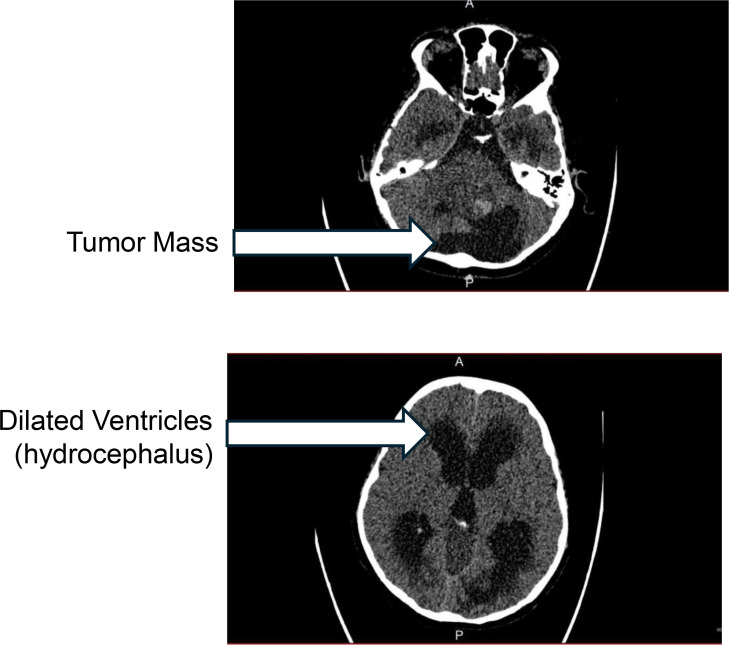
Non-contrast axial CT of the brain at presentation (age 14) demonstrating a posterior fossa mass occupying the fourth ventricle (arrow) with moderate supratentorial hydrocephalus (arrow). The mass is confined to the posterior fossa with no supratentorial extension or involvement of the anterior visual pathways. CT, computed tomography.
